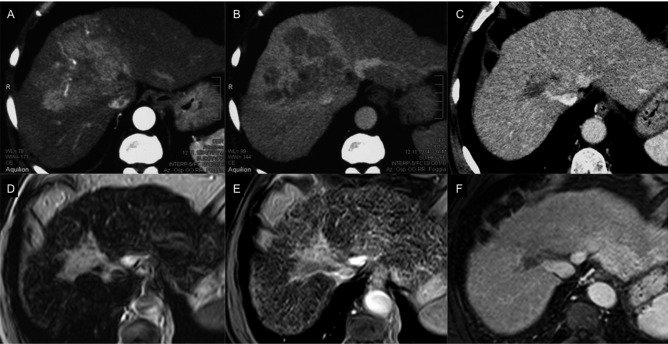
(A and B) Axial computed tomography (CT) scans at the baseline in arterial (A) and delayed (B) phases revealing a hypervascular mass in hepatic segment VIII. (C) Axial CT scan following 30 months of sorafenib therapy in the portal venous phase, revealing a hypodense lesion with a significant size reduction. (D and E) Double-contrast MRI scan following 35 months of sorafenib therapy, revealing a barely hyperintense lesion in a T2-weighted image after hepato-specific superparamagnetic iron oxide (SPIO) contrast agent administration (D) and a homogeneous lesion enhancement in a T1-weighted image after gadolinium administration (E), resembling fibrotic tissue. (F) MRI performed after 48 months of therapy, revealing substantial stability of the disease in a T1-weighted image following gadolinium administration.
